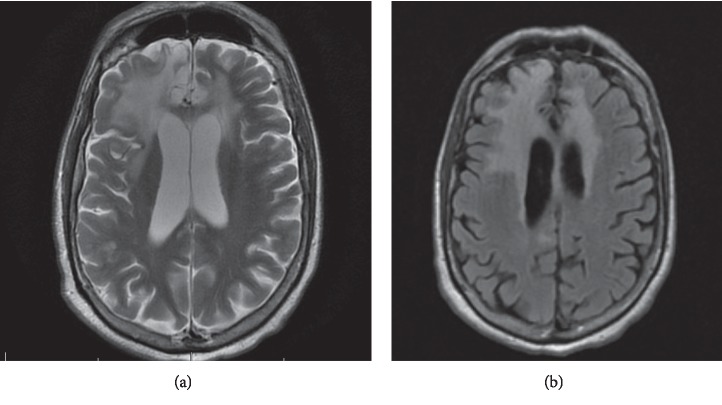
MRI brain after 11 months of HSVE. T2 (a) and T2/FLAIR (b) showed no additional changes other than the interval evolution of areas of encephalomalacia in right frontotemporal lobes.
